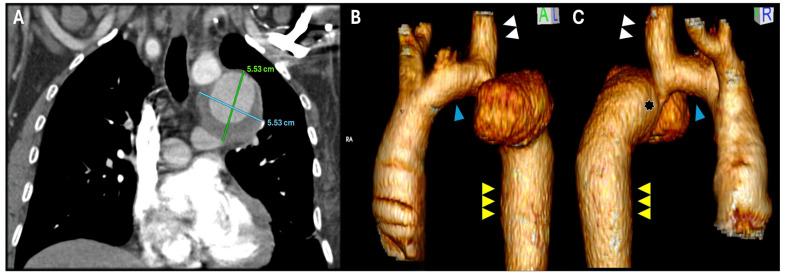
Preoperative CTA (A) and 3D reconstruction (B,C) showing the post-coarctectomy aneurysm on the distal segment of the aortic arch. Hypoplasia of the proximal aortic arch ((B,C), blue arrowhead), hypertrophy of the LSA ((B,C), white double arrowhead) and dilation of the distal thoracic aorta ((B,C), yellow triple arrowhead) can be identified. Re-coarctation signs are depicted ((C), asterisk) compatible with the preoperative pressure gradient.
